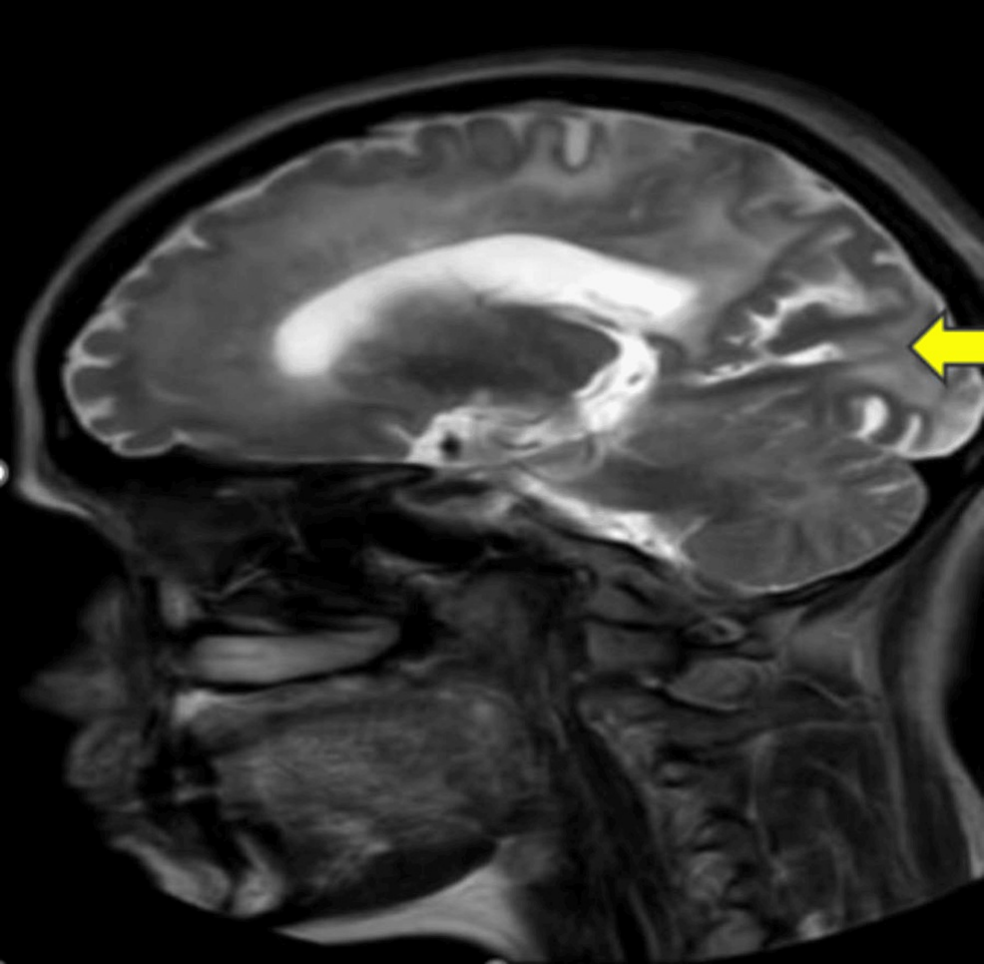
MRI of the brain revealed leukoencephalopathy (CADASIL) (yellow arrow) MRI: magnetic resonance imaging, CADASIL: cerebral autosomal dominant arteriopathy with subcortical infarcts and leukoencephalopathy
